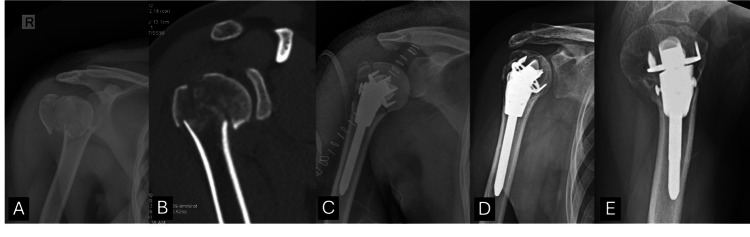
(A) Preoperative X-ray. (B) Preoperative CT image. (C) Immediate postoperative X-ray AP view. (D, E) Final follow-up X-rays AP and lateral views at 12 months showing united fracture.
